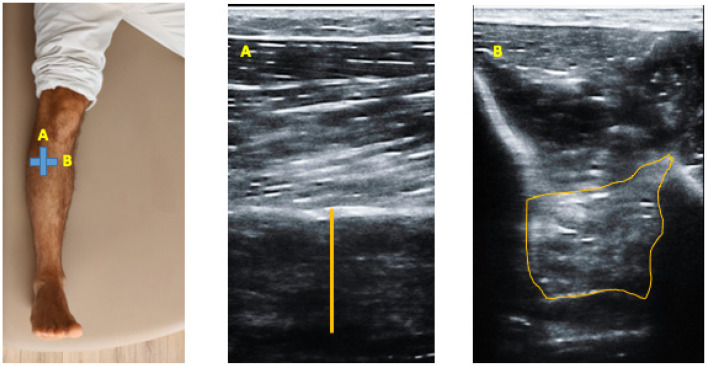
Ultrasound imaging thickness and CSA of the TP muscle. (A) Upper third of the imaginary line in a longitudinal view (thickness) and (B) Transversal view (CSA) to identify the TP.
